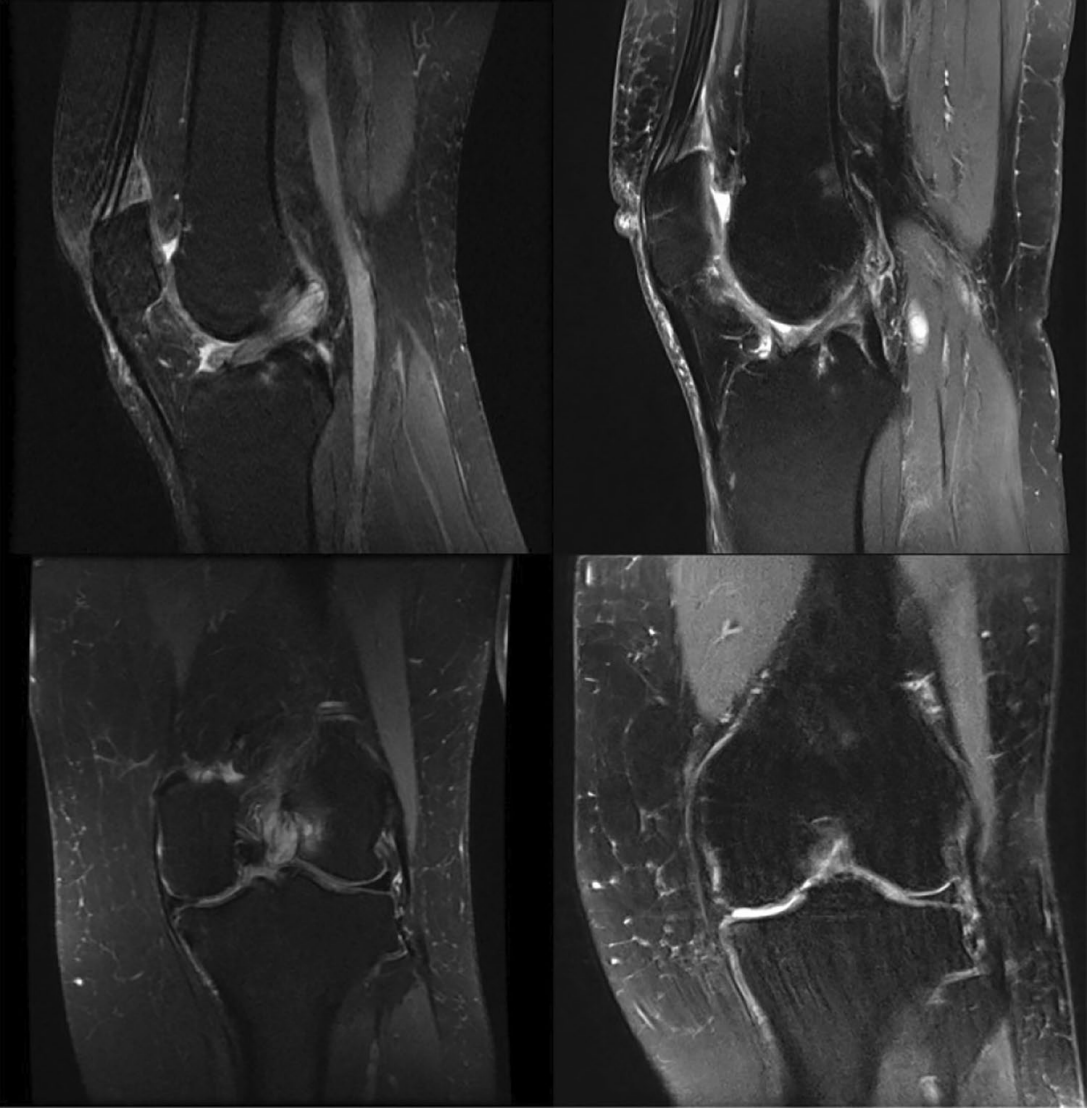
MRI of MDACL before and after treatment of partial resection
